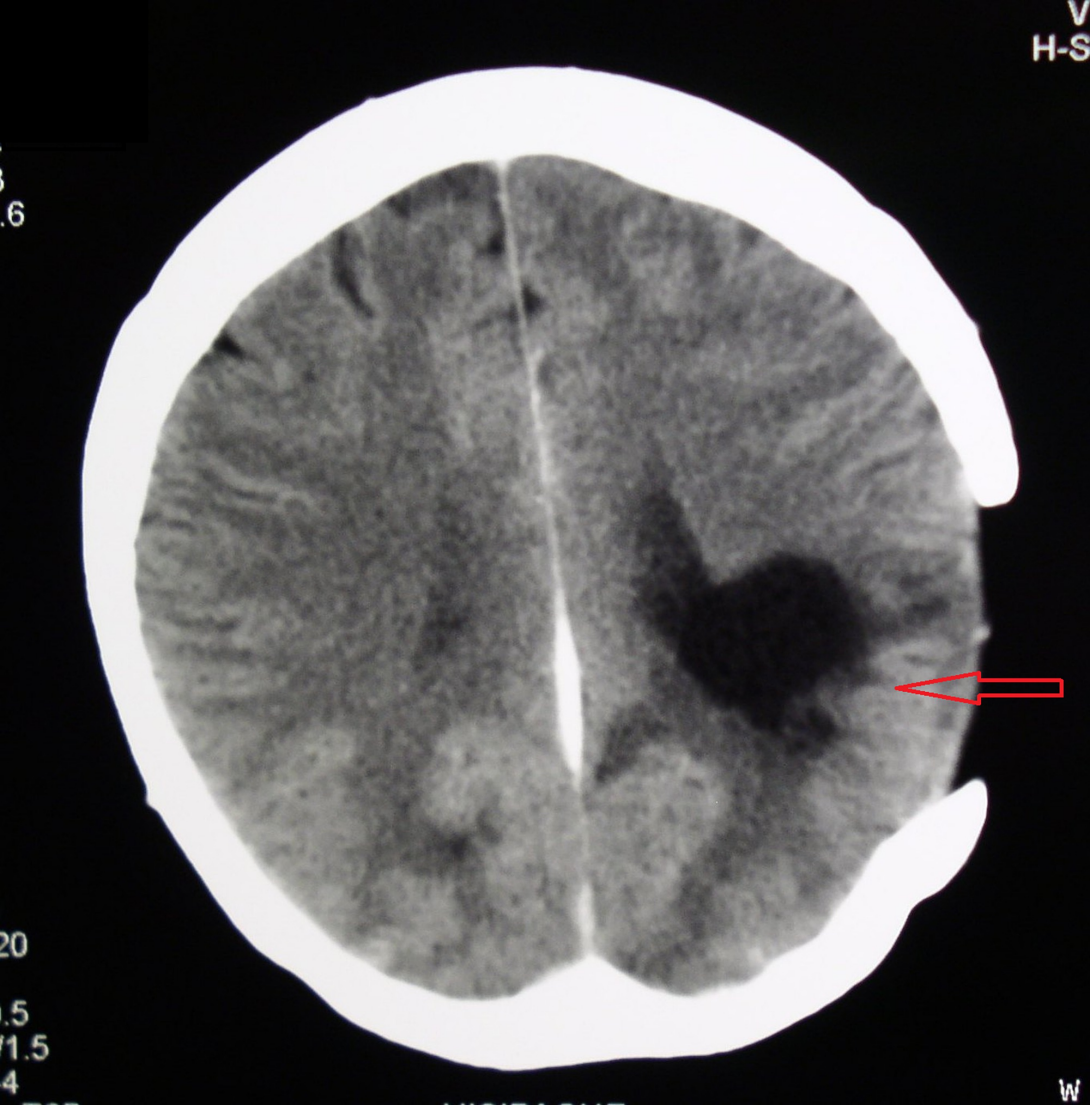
A CT scan obtained two months after the second re-surgery for the abscess. A porencephalic cyst after the abscess removal is visible. During the discharge from the hospital the patient has been without a neurological deficit.
